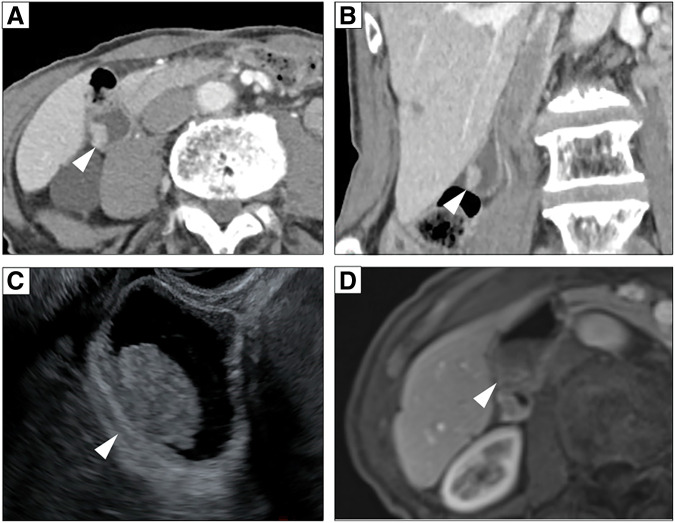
Preoperative imaging. Enhanced computed tomography and T2-weighted magnetic resonance imaging and endoscopic ultrasonography. (A, B) Contrast-enhanced computed tomography showed a nodular lesion at the fundus of the gallbladder (arrowheads). (C) Endoscopic ultrasound showed a protruding lesion of 22 mm at the fundus of the gallbladder and which had sub-serosal invasion (arrowhead). (D) Gd-EOB-DTPA enhanced magnetic resonance showed a nodular tumor at the fundus of the gallbladder without evidence of lymph node or distant metastasis (arrowhead).
